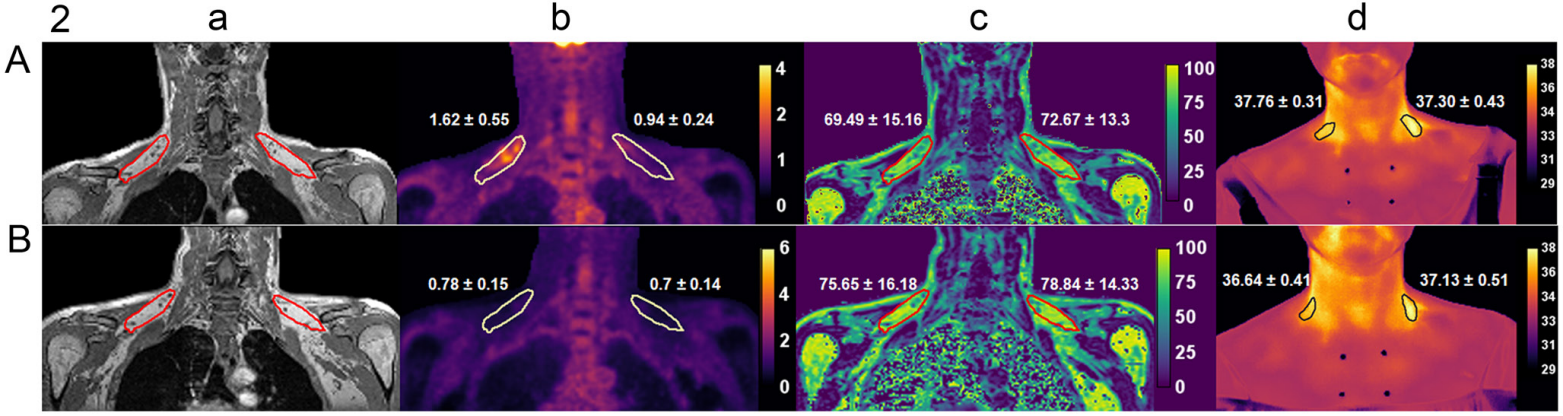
Representative images highlighting supraclavicular brown adipose tissue (sBAT) from a BAT-positive subject (A) and a BAT-negative subject (B) at hyperthyroidism (top) and euthyroidism stage (bottom). (a) T1-weighted anatomical MR image sBAT, (b) ^18^F-FDG PET (c) the fat fraction (FF) map. (d) IRT map with color bar showing the temperature in °C. The PET, FF, and IRT maps in which color scale shows values of standardized uptake values (SUV), FF (in %), and Tscv, respectively. The regions of interest (ROI) drawn around sBAT on the anatomical MR images, PET, FF, and IRT maps showing the location of sBAT. The values (mean ± s.d.) of SUV, FF, and Tscv are shown of the corresponding region of interest.
